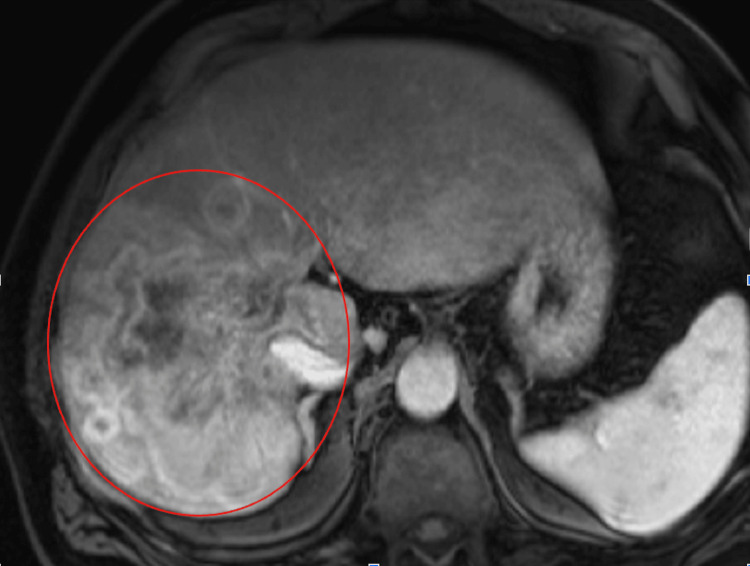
Axial view of the MRI abdomen demonstrating a large mass in the central aspect of the right lobe of the liver measuring approximately 12.0 x 9.7 x 8.6 cm extending into the caudate lobe and abutting the inferior vena cava (IVC)
